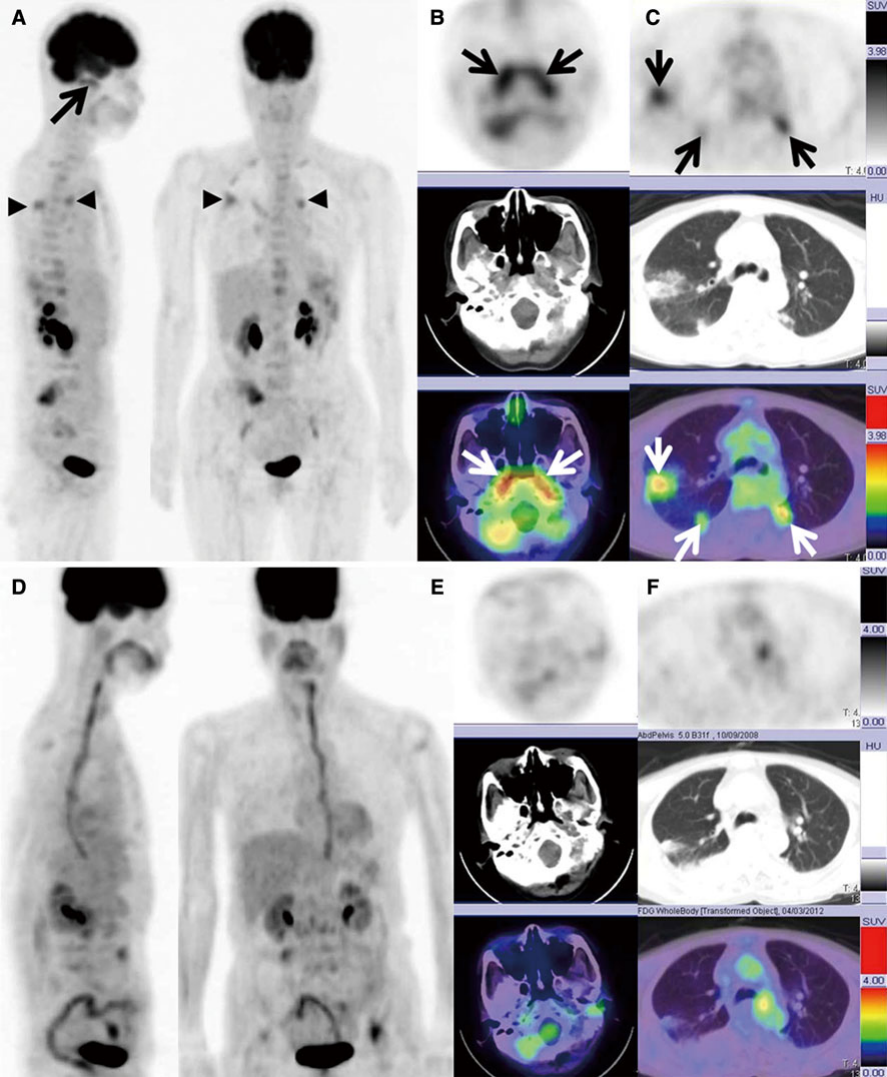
A 65-year-old woman (Case 3) with WG underwent PET/CT. Serum MPO-ANCA titer and PR3-ANCA titer were elevated to 27.4 and 5.5 U/ml, respectively. a An MIP image showing the abnormal FDG uptake in the nasopharynx (*arrow*) and lung (*arrow heads*). b An axial FDG PET/CT image showing increased FDG uptake in the bilateral auditory tubes (*white and black*
